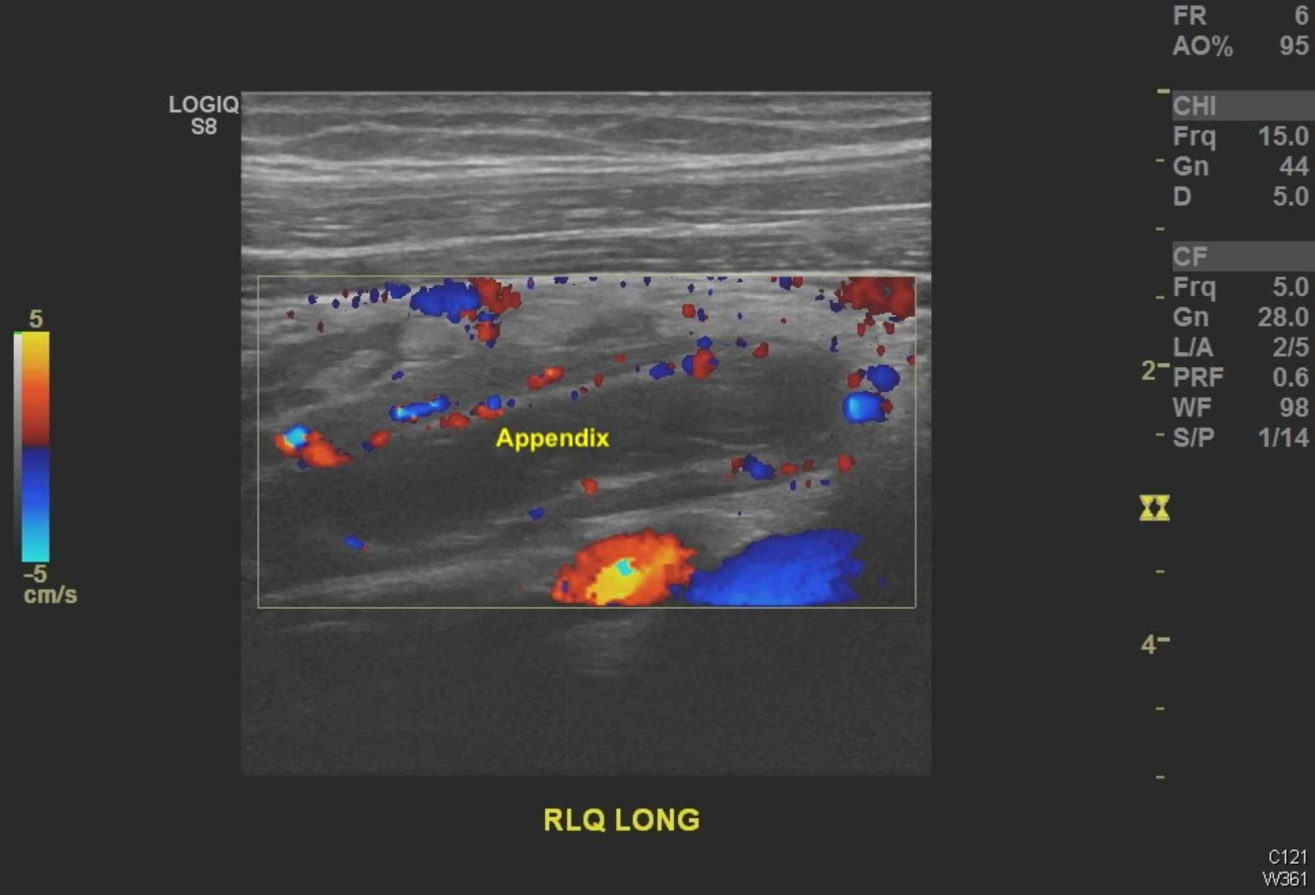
Sonogram demonstrating a long axis view of the appendix, a tubular structure that appears elongated beyond normal.
